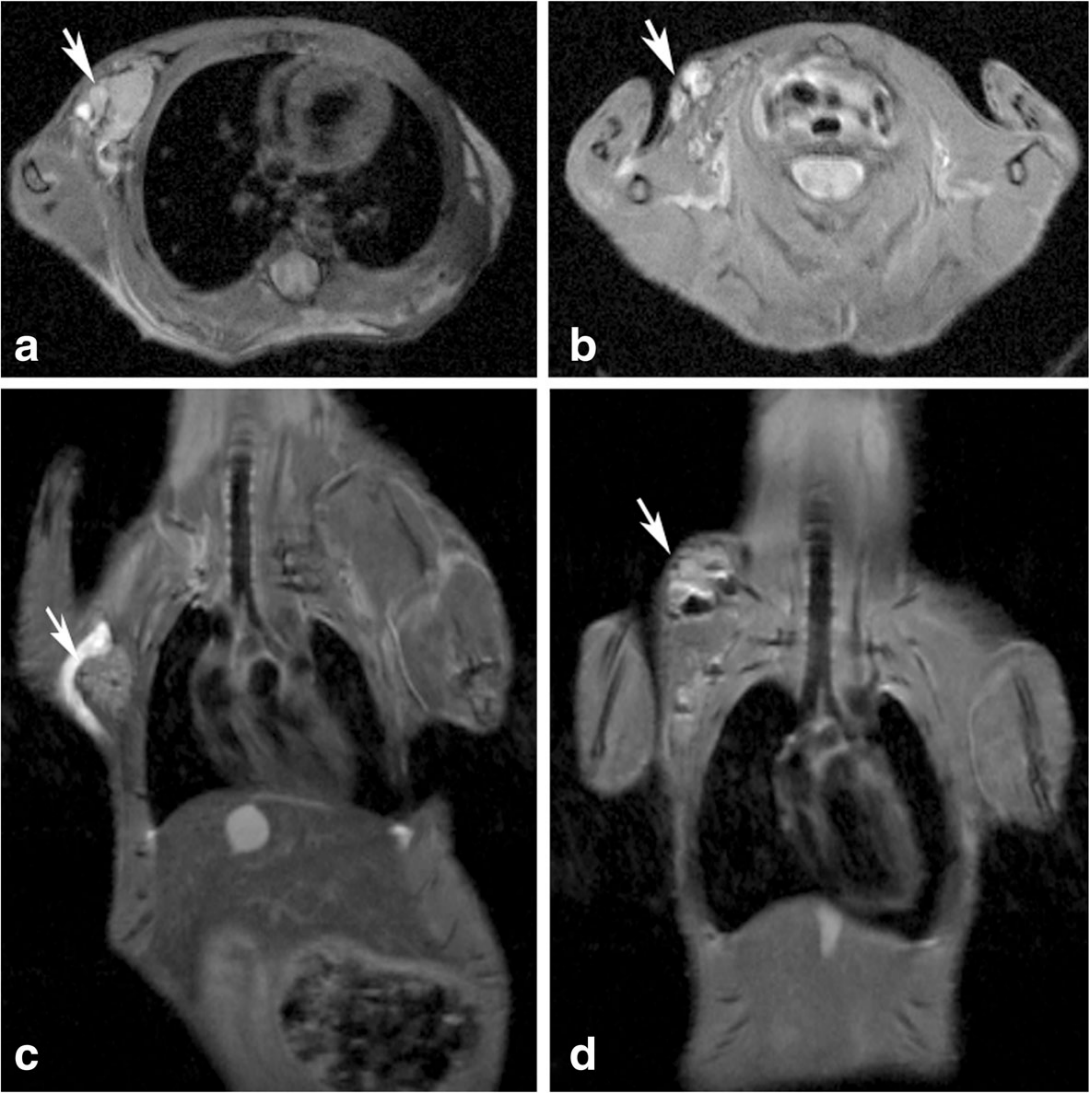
Magnetic resonance imaging of two transplanted tumors. a and c showed the horizontal plane and coronal plane of one transplanted tumor, respectively, b and d showed the horizontal plane and coronal plane of the other transplanted tumor. The tumors in the dorsal flank were pointed out by arrows
